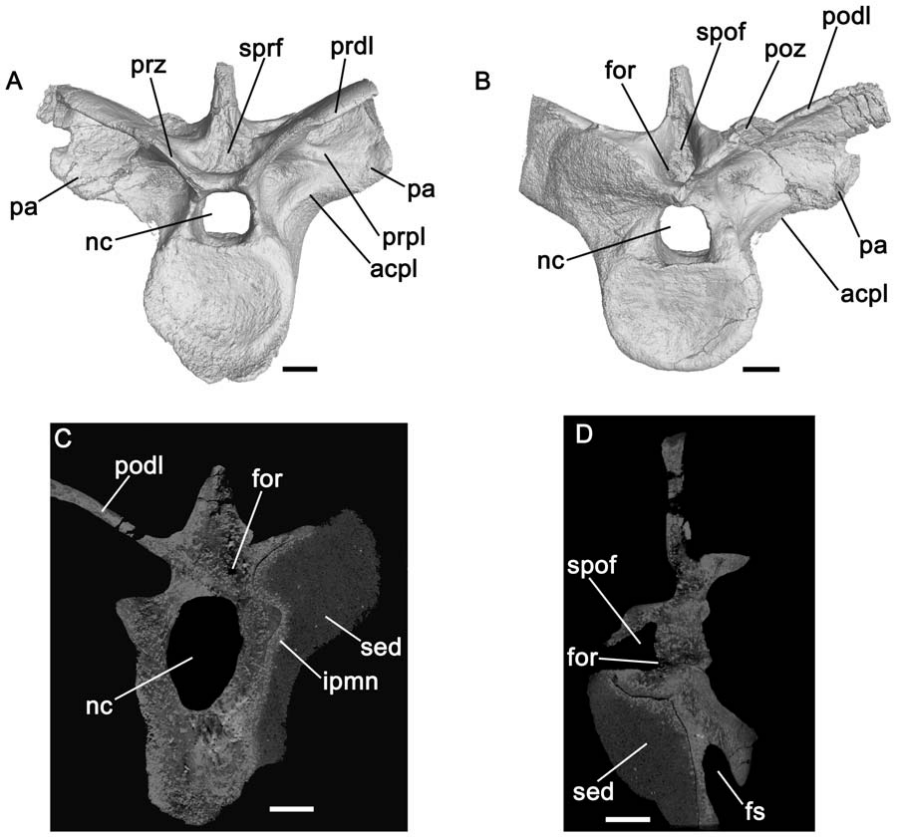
Paratypothoracine aetosaur, anterior dorsal vertebra (NHMUK OR38070). A, B: dorsal vertebra in anterior (A) and posterior (B) views. Note that there is a large volume of sediment adhering to the posteroventral surface of the left transverse process. The left transverse process was incompletely scanned and so is artificially truncated at a point just distal to the parapophysis. C: CT slice showing transverse section (in anterior view) immediately anterior to the base of the postspinal fossa. The left of the two foramina within the postspinal fossa is visible, and is surrounded by dense trabecular bone. D: CT slice showing section through the neural canal. The position of the left foramen within the postspinal fossa is marked. Note that in both CT slices intertrabecular spaces are mineral-infilled; pore spaces in the sediment immediately adjacent to the external surface of the bone are also infilled. Abbreviations: acpl, anterior centroparapophyseal lamina; for, foramen; ipmn, mineral-infilled pore spaces within sediment adjacent to the bone; nc, neural canal; pa, parapophysis; podl, postzygodiapophyseal lamina; poz, postzygapophysis; prdl, prezygadiapophyseal lamina; prpl, low ridge forming incipient prezygaparapophyseal lamina; prz, prezygapophysis; sed, sediment; spof, spinopostzygapophyseal fossa; sprf, spinosprezygapophyseal fossa. All scale bars equal 10 mm.
